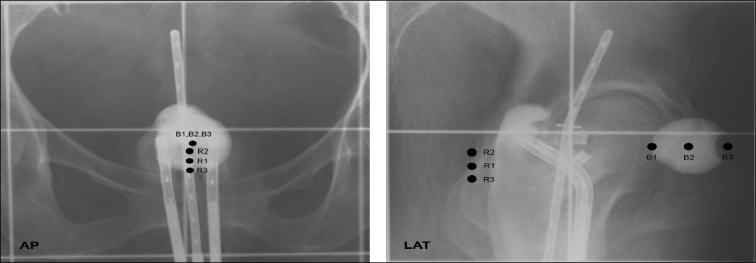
Selection of Rectal and Bladder Points in AP and LAT radiographs
